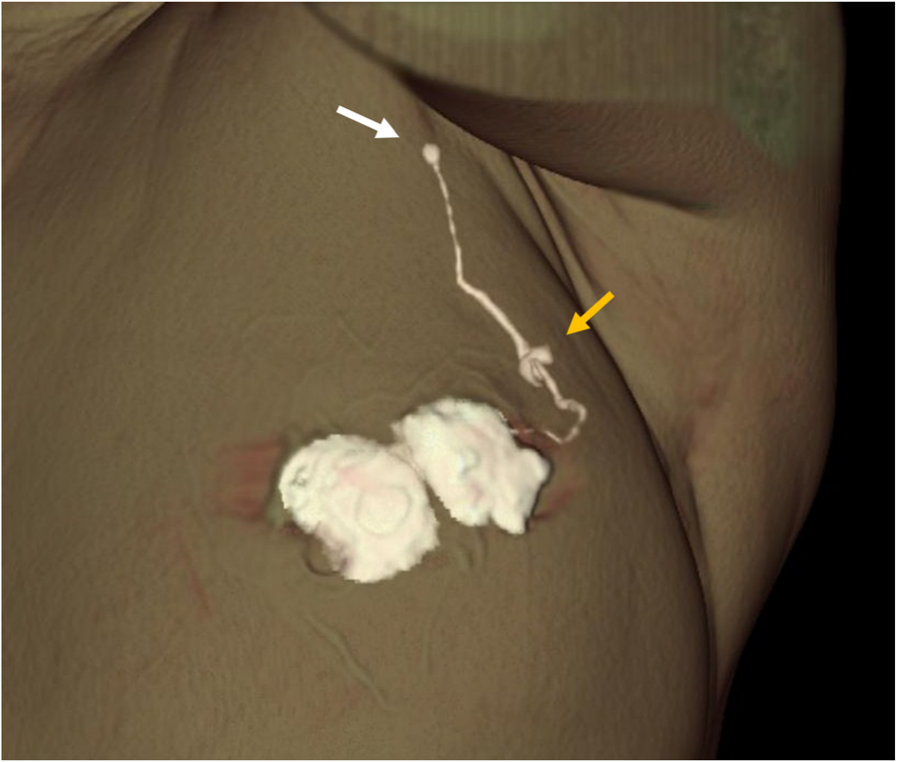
Three-dimensional computed tomography-lymphography (CT-LG) reconstructed from the first post-contrast images. Contrast agent was injected intradermally into the skin overlying the breast tumor and the subareolar skin. Lymphatic vessels drained into a single axillary sentinel node (yellow arrow). CT-LG can visualize lymph flow and can distinguish sentinel nodes from non-sentinel nodes (white arrow).
